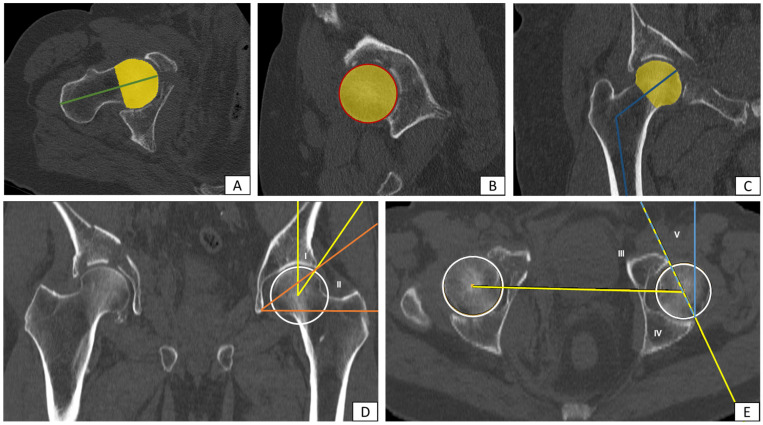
Example of the measurement of proximal femur and acetabular geometry using CT data with multiplanar reconstruction: (A) femoral neck length (green), (B) femoral head diameter (red), (C) real CCD angle (blue), (A–C) femoral head volume (yellow), (D) (I) CE angle mod. acc. to Wiberg (yellow), (D) (II) rotational angle mod. acc. to Ullmann (orange), (E) (III) anterior acetabular sector angle (yellow/AASA), (E) (IV) posterior acetabular sector angle (yellow/PASA), (E) (V) acetabular anteversion acc. to Anda (blue).
